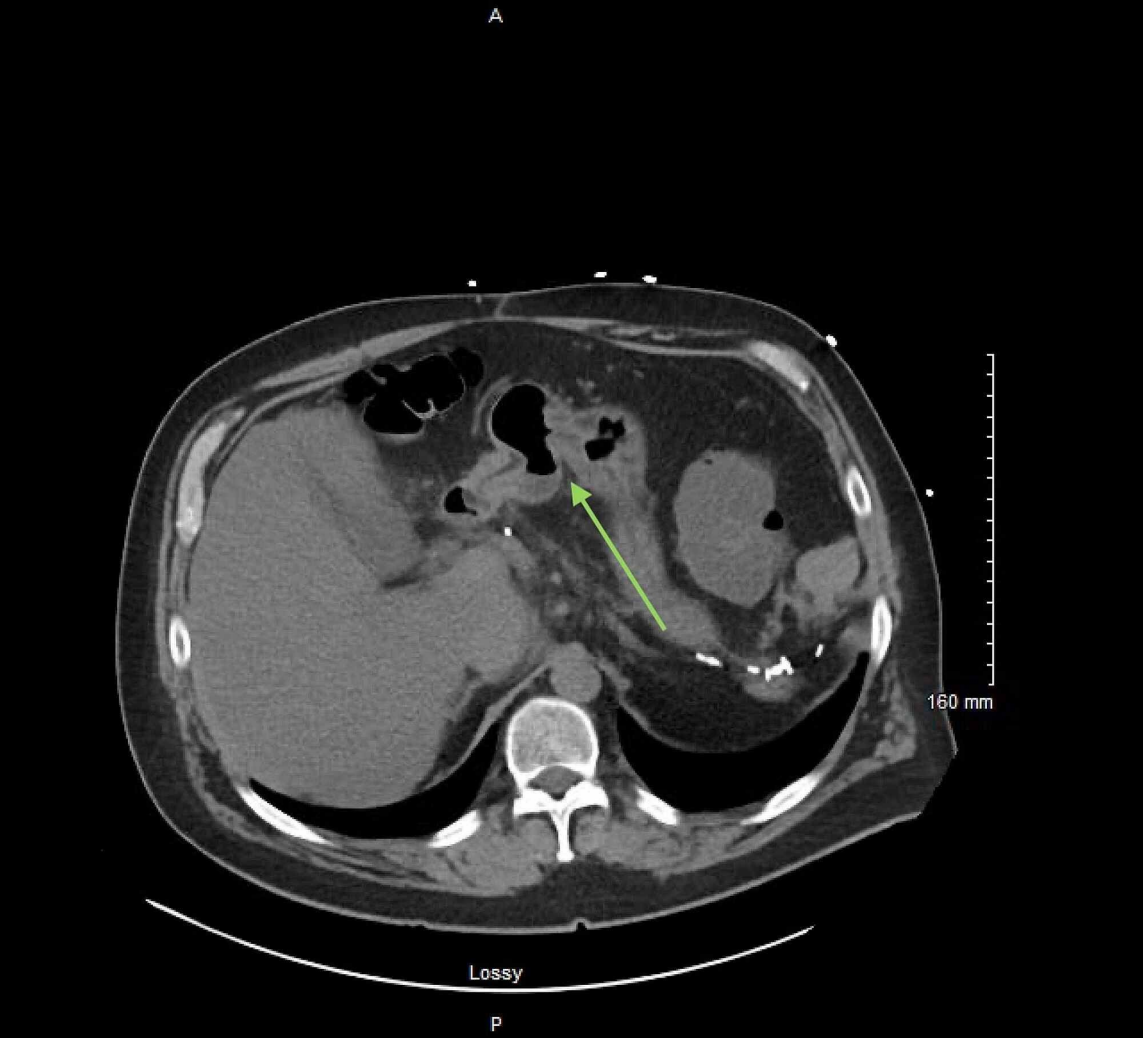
Unenhanced cross section CT scan of the abdomen showing an ileocolonic fistula with aneurysmal dilation of the small bowel (green arrow).
